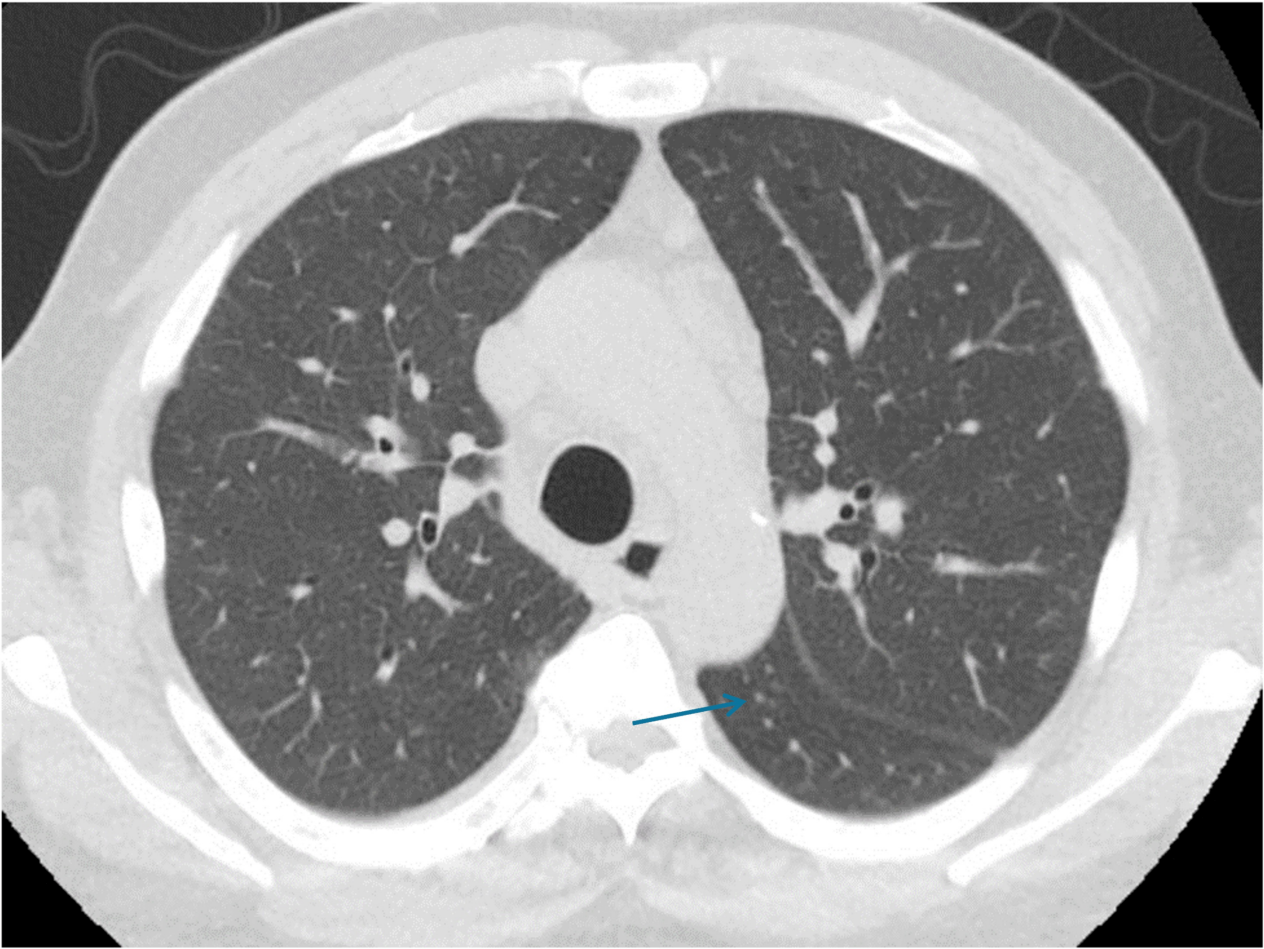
Follow-up CT scan of the chest Interval resolution of previously seen airspace disease within LUL. Numerous mediastinal lymph nodes, many of them are subcentimeter in size.
